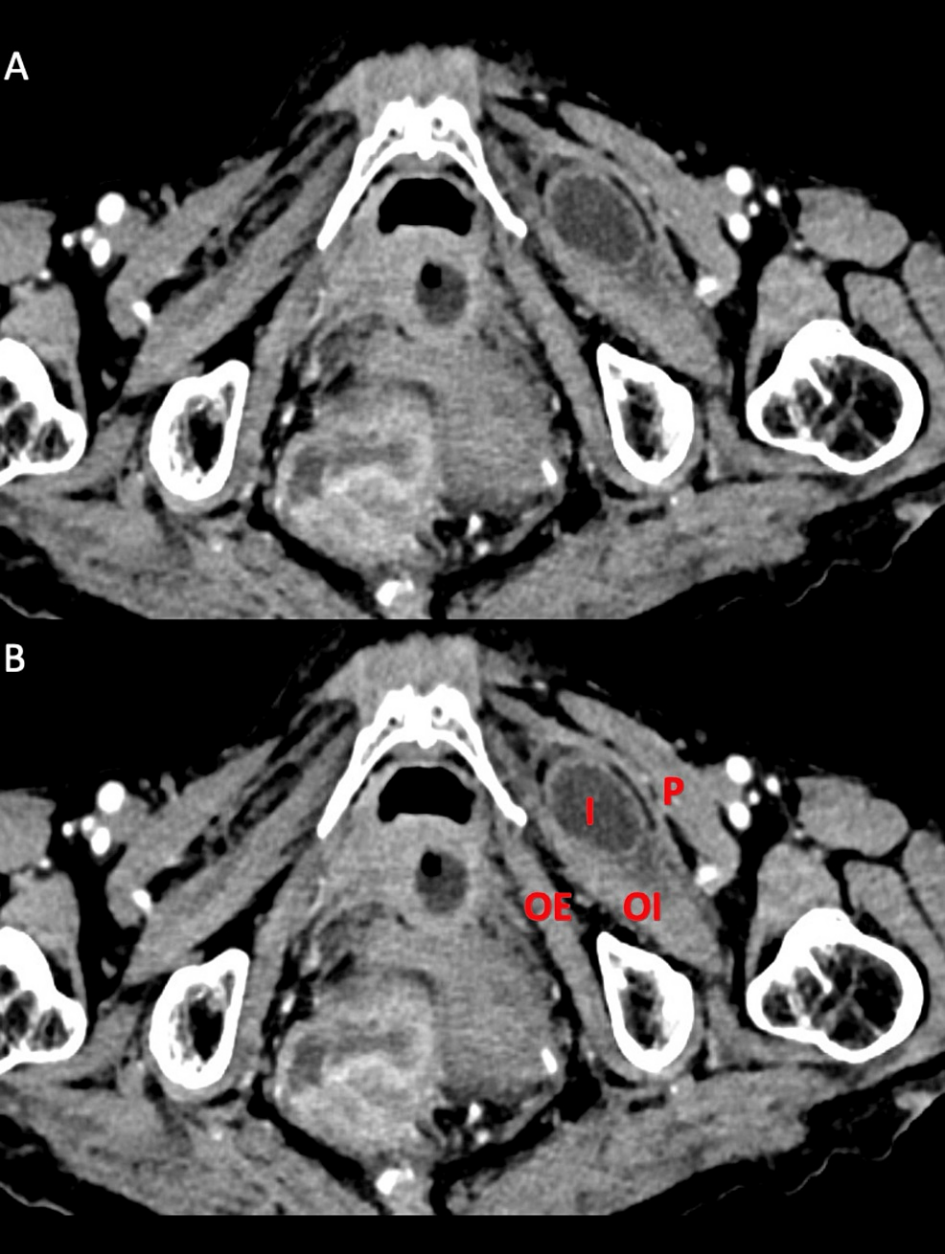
Contrast-enhanced CT showing obturator hernia with bowel as content Contrast-enhanced CT images of the abdomen in a case of intestinal obstruction. Axial (A-unmarked, B-marked) images show the left-sided obturator hernia with a dilated ileal loop (I) lying in between the obturator internus (OI) and pectineus (P) muscles. OE - obturator externus muscle
